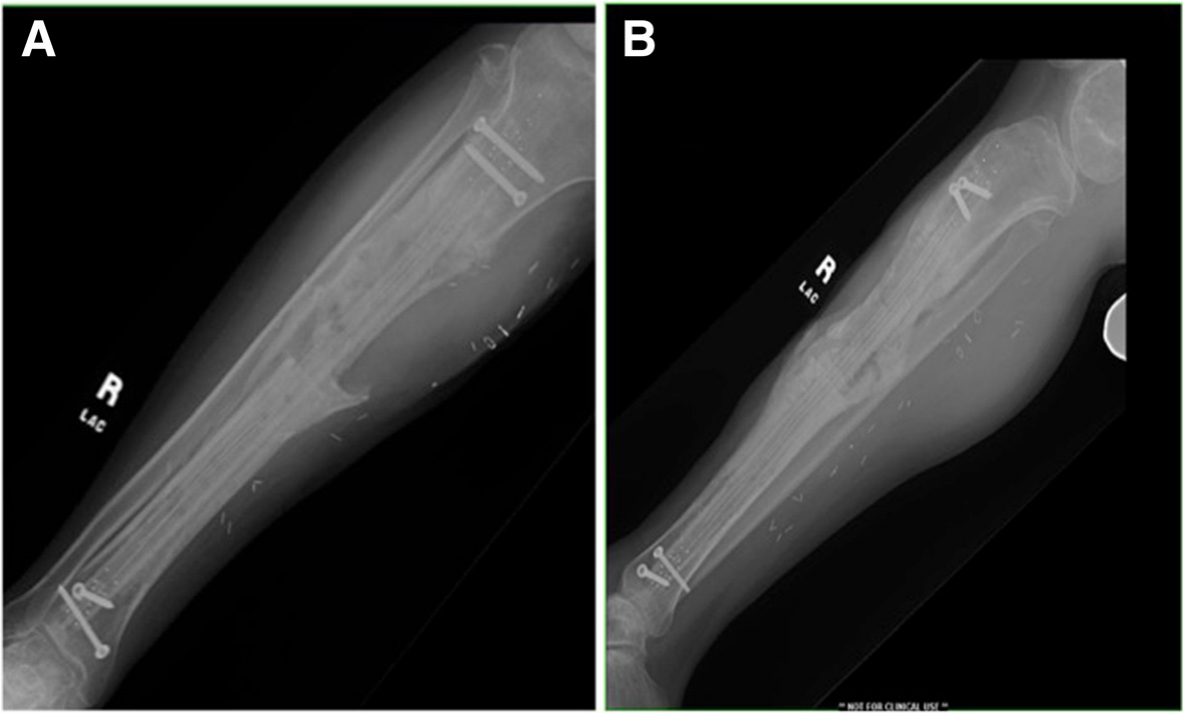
Standard AP (a) and lateral (b) plain radiograph taken at 5 months postoperative follow-up demonstrating improved bone healing without evidence of osteolysis, infection, or hardware migration
